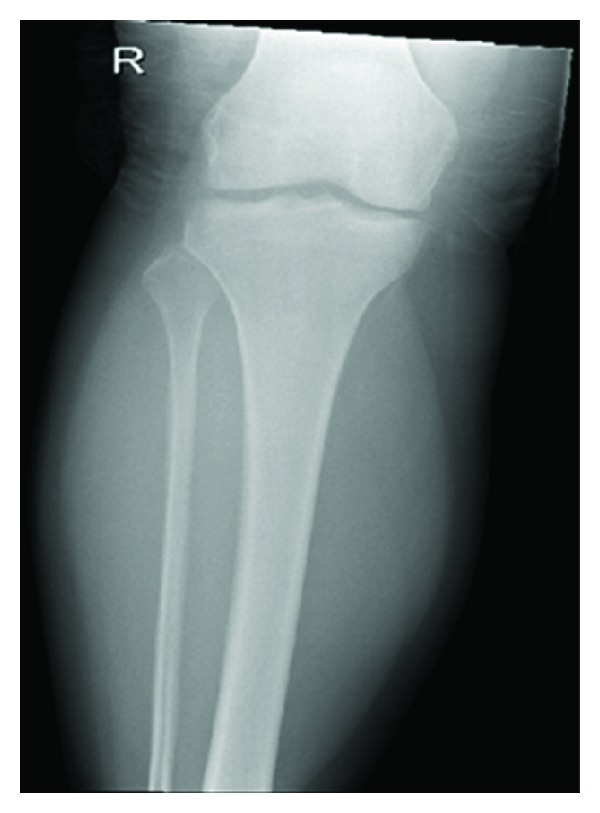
AP plain X-ray of right lower leg showing no abnormality.
